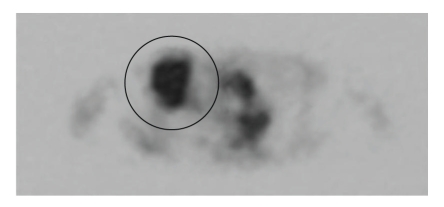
FDG-PET at 13 months after SBRT. High FDG uptake (SUVmax  = 5.61) was observed for the consolidation.
